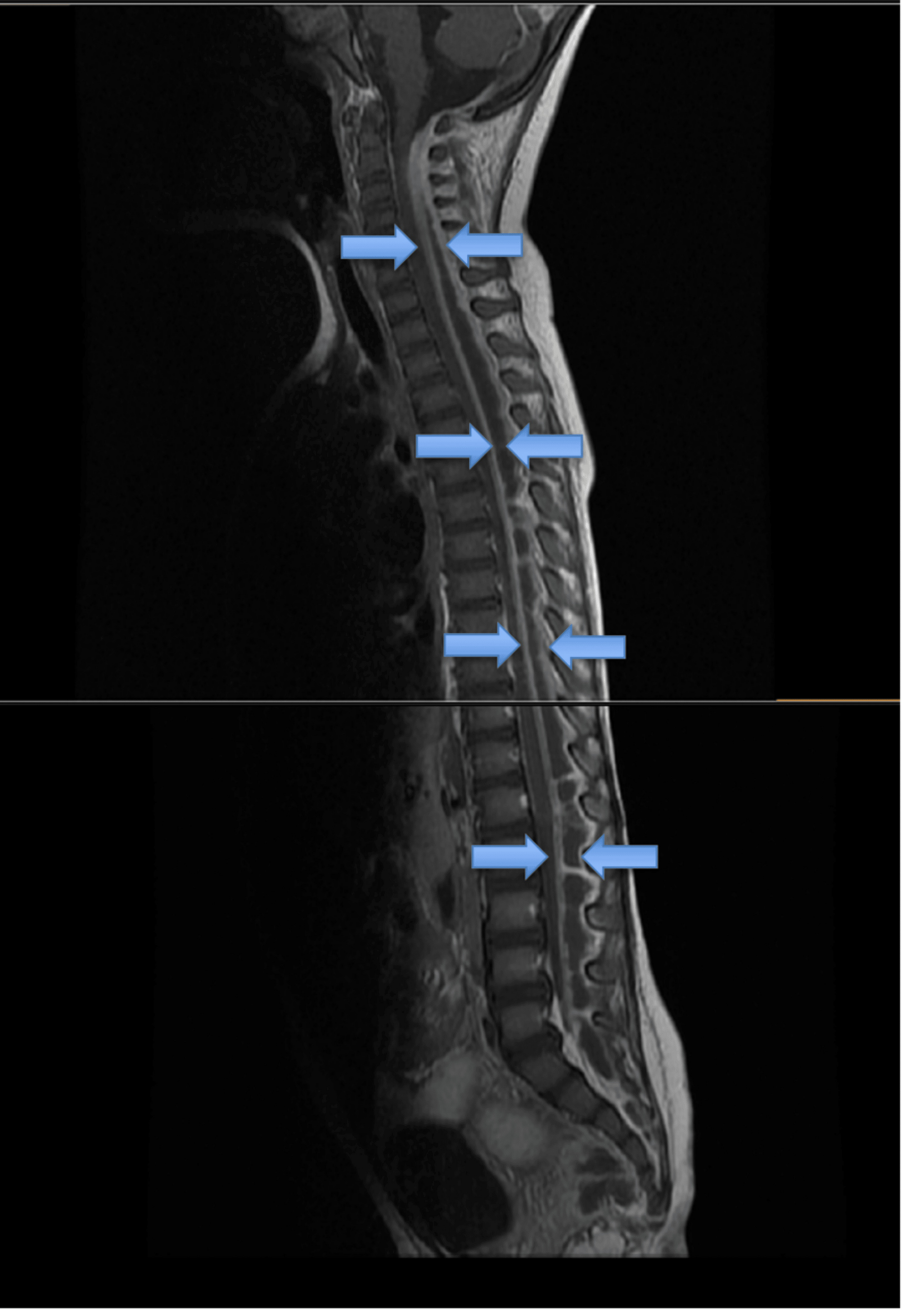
MRI at presentation (day one). Contrast-enhanced sagittal T1-weighted MRI depicting a holospinal epidural abscess extending from the cervical to the sacral spine, compressing the spinal cord.
